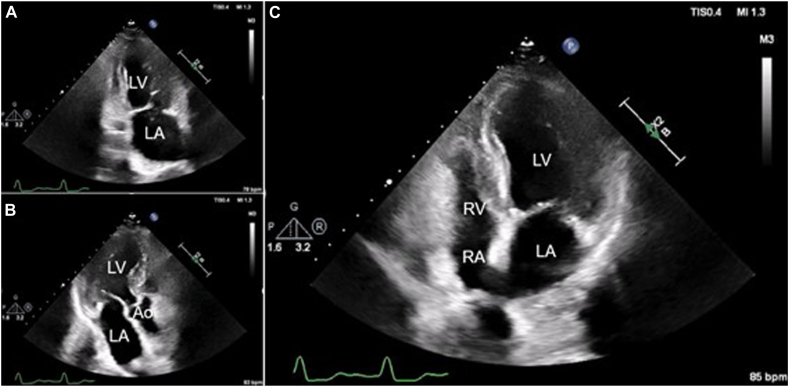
Two-dimensional TTE, apical 2-chamber (A), apical 3-chamber (B), and apical 4-chamber (C) systolic views performed 2 years earlier, demonstrates normal left ventricular and left atrial size without visualized mass. *LV*, Left ventricle; *RA*, right atrium; *RV*, right ventricle.
